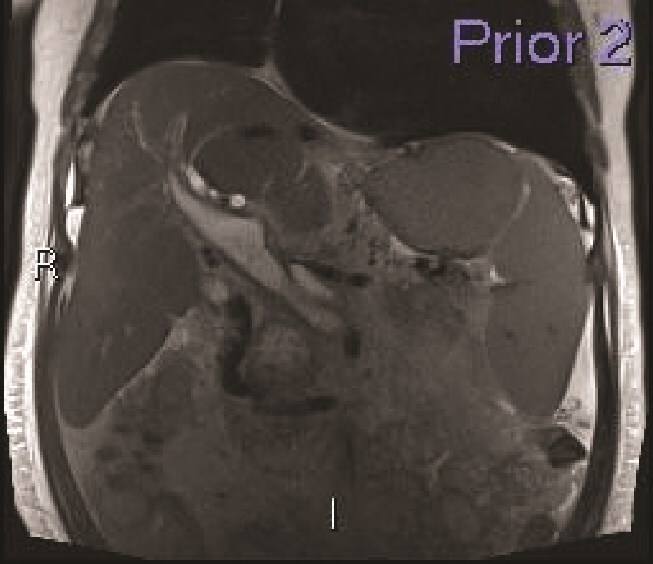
MRI—Coronal reconstruction. The main portal vein and its intrahepatic branches show loss of normal T2 signal void and instead shows distension with T2 hyperintensity within, matching the signal of the pancreatic neck collection (not shown). There is mild bi-lobar intrahepatic biliary radicle dilatation.
